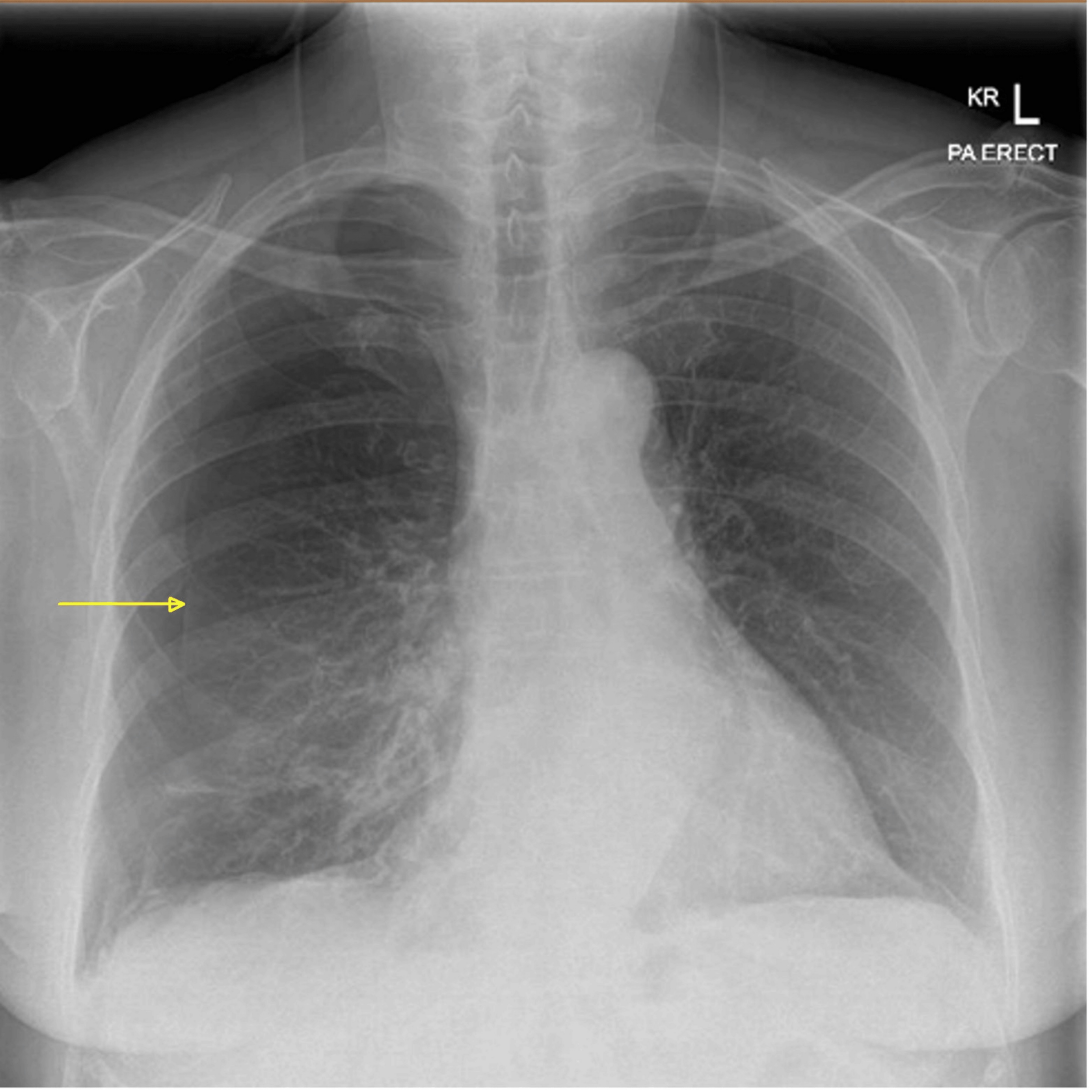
Posteroanterior erect chest X-ray on admission The image shows a right-sided pneumothorax, with the arrow pointing to the lung edge
